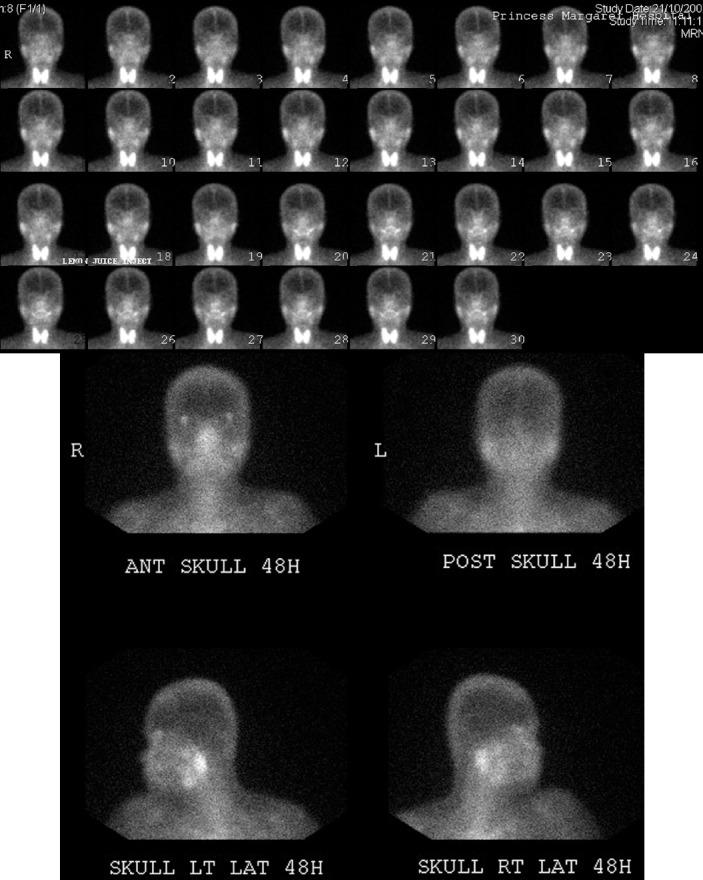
Images of a 60-year-old woman (Patient 11). (A) ^99m^Tc-pertechnetate scintigraphy shows decreased and minimal accumulation of tracer in parotid glands and submandibular glands, respectively. Both parotid glands show normal excretory function after stimulation (lemon juice at 15 minutes). (B) Gallium scan shows normal uptake in the bilateral parotid glands. No abnormal uptake is noted in the lacrimal gland
